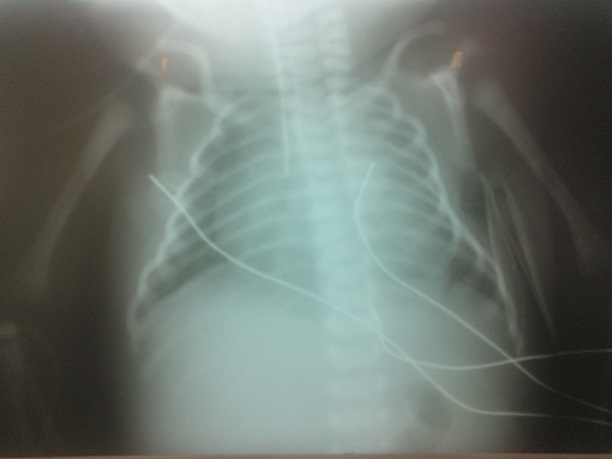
Chest radiograph showing an enlarged cardiac silhouette
